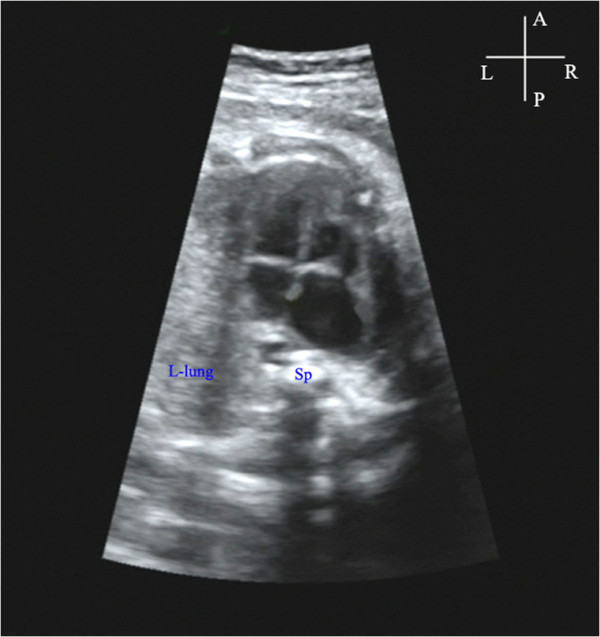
Ultrasonographic image of a 35-gestational-week fetus diagnosed with right lung agenesis. At the transverse plane of the thorax level, a right-sided heart beside the left lung was demonstrated and the cardiac axis was about 50° toward right side. Sp, spine.
